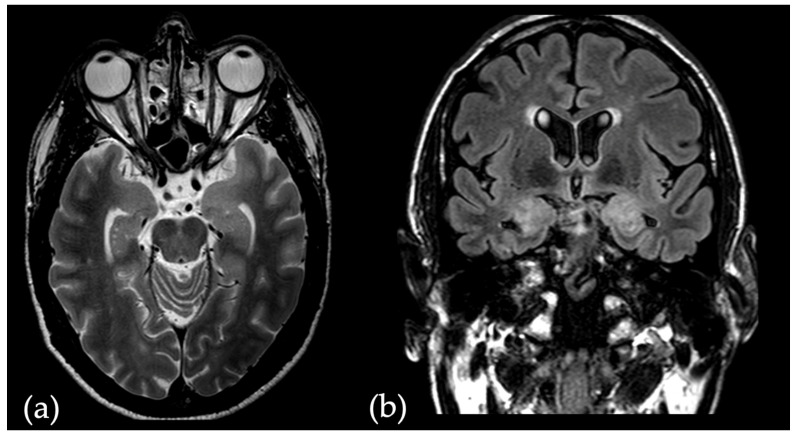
(a) T2-image of a 54-year-old seropositive AE patient who presented with serial seizures. Left-leading hyperintensity and enlargement of the mesial temporal lobe. (b) Corresponding fluid attenuated inversion recovery (FLAIR) sequence in the coronal plane showing bilateral hyperintensity of the mesial temporal lobe.
